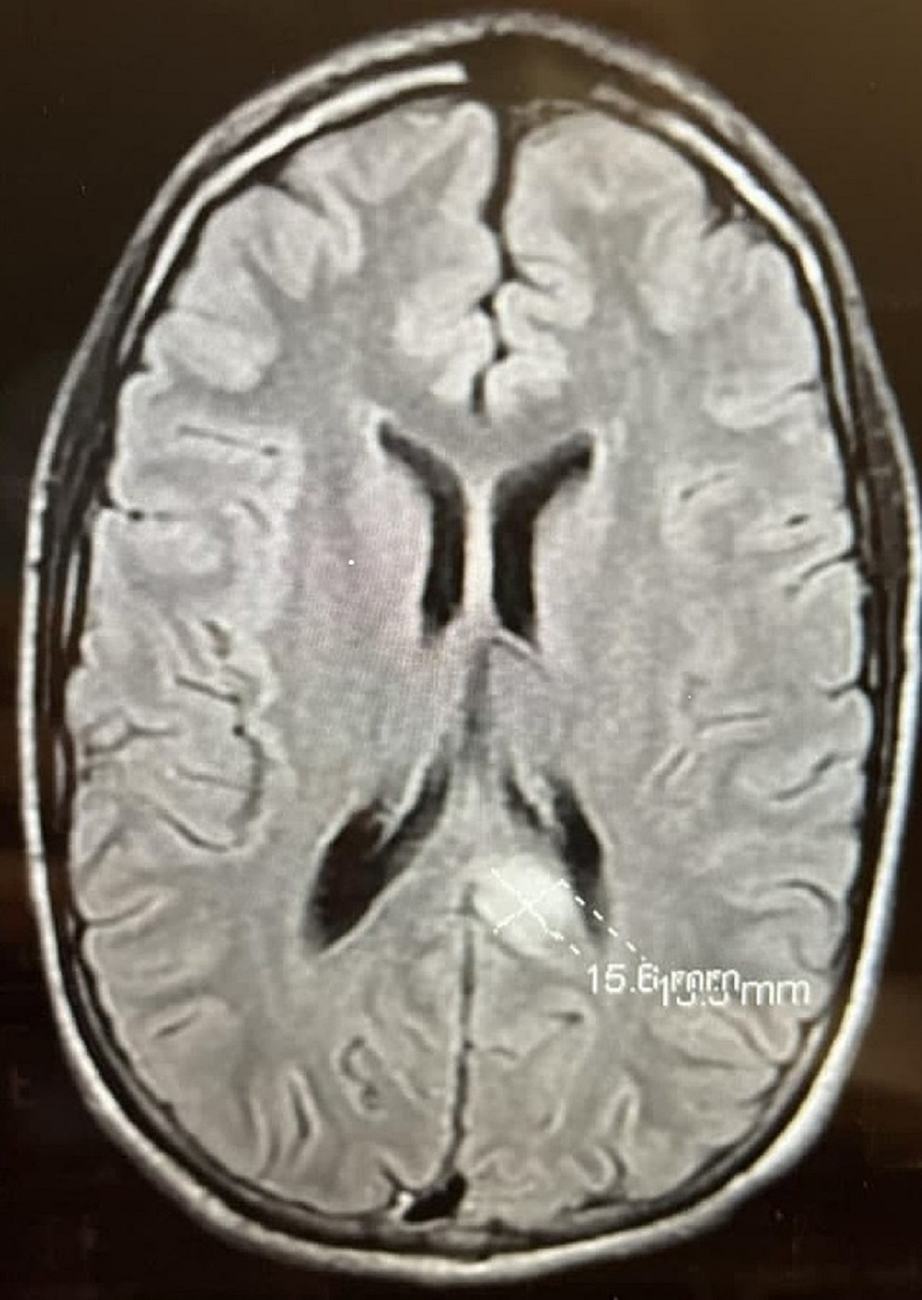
MRI brain with contrast showing enhancement of the splenial lesion MRI: magnetic resonance imaging
